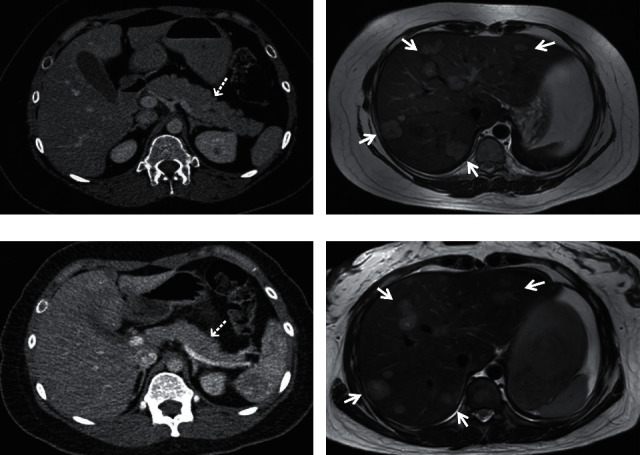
Abdominal CT and MRI images before (a and c) and after 7 cycles of octreotide LAR therapy (b and d) (case 1). (a) The abdominal CT image demonstrated a solid nodule in the body-tail junction of the pancreas (dotted arrow). (b) The abdominal MRI demonstrated multiple blood-rich nodules in the liver (arrow). (c) The abdominal CT image demonstrated that the pancreatic solid nodule was unclear after 7 cycles of octreotide LAR therapy (dotted arrow). (d) The abdominal MRI demonstrated that the sizes and numbers of hepatic metastatic nodules remained stable (arrow). CT: computed tomography. MRI: magnetic resonance imaging.
